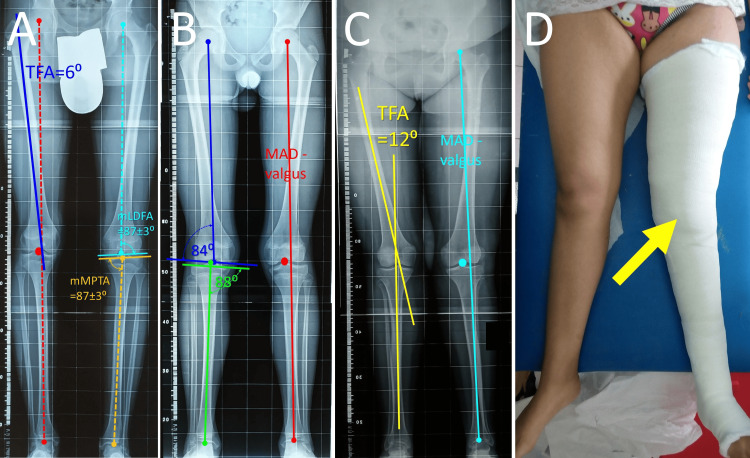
Mechanical axis assessment on ortho-roentgenogram of the lower limbs (A) Normal ortho-roentgenogram. The red dotted line is the mechanical axis of the lower limb. The dark blue line is the anatomical axis of the right femur. The mLDFA (mechanical lateral distal femoral angle) is the angle formed between the mechanical axis of the femur (dotted light-blue line) and the tangential to the femoral condyles line (continuous light-blue line). The mMPTA (mechanical medial proximal tibial angle) is the angle formed between the mechanical axis of the tibia (orange dotted line) and the tangential to the tibial condyles (continuous orange line). (B) A patient with recurrent PD. The mechanical axis of the lower limb (red line) is lateral to the center of the knee (red dot) indicating a valgus mechanical axis deviation (valgus-MAD) of the knees. Measurement of the mLDFA and the mMPTA indicate that the distal femur is the predominant cause of MAD. (C) A patient with acute lateral PD. The tibiofemoral angle (TFA) is the angle between the anatomical axis of the femur and the tibia (yellow lines). It is also useful to quantify knee valgus. A valgus-MAD is evident as well (light-blue line and dot). (D) The patient of Figure C. Marked knee-valgus is obvious on clinical examination. The patient received acute repair of a patellar avulsion of MPFL, and the knee was immobilized in a long-leg cast (yellow arrow). A, B, C: Figures created by Panagiotis Samelis. D: Figure published under Creative Commons Attribution License CC-BY 3.0 [40].
